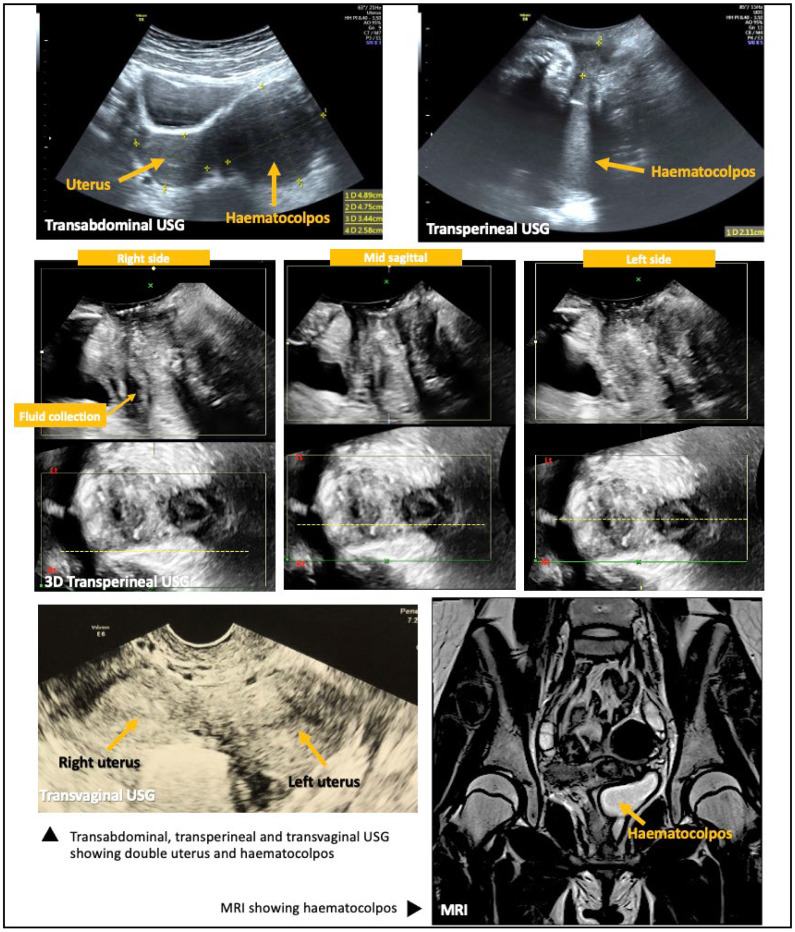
Ultrasound and MRI of cases with OHVIRA.
